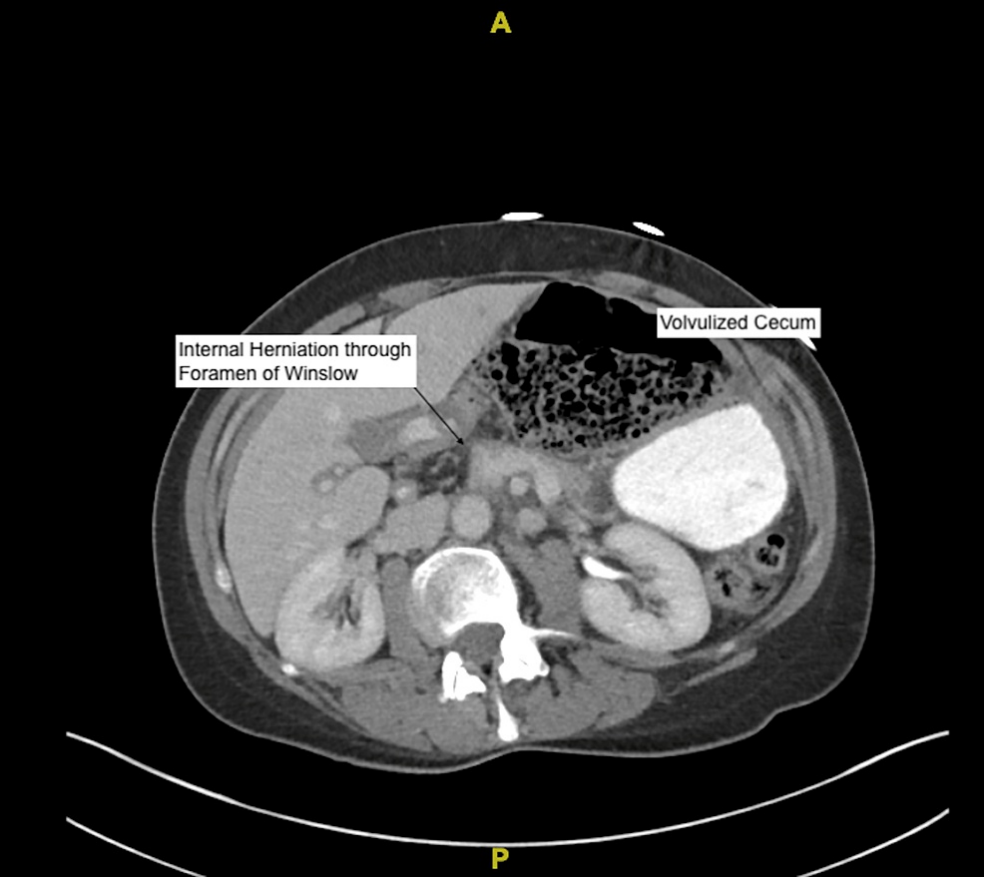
CT of the abdomen showing the cecum herniating through the Foramen of Winslow.
